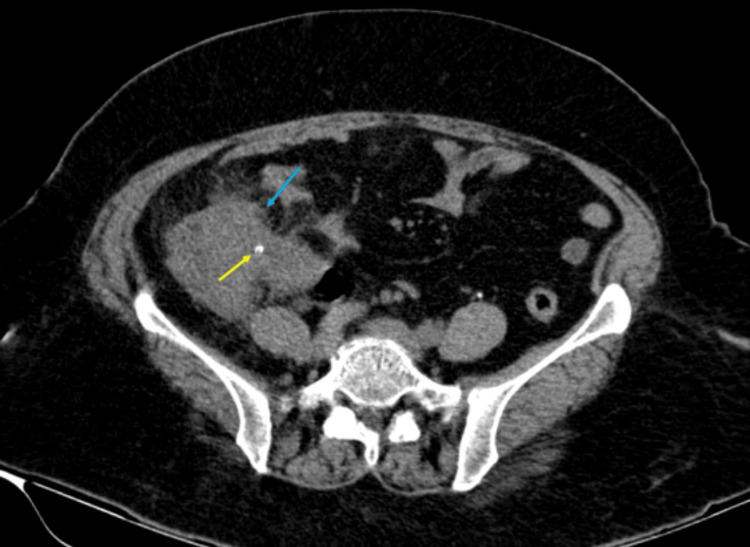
CT of the abdomen axial section showing appendicular mass with perforation (blue arrow) and tiny hyper-dense focus within the appendicular mass (yellow arrow). CT: Computed tomography
